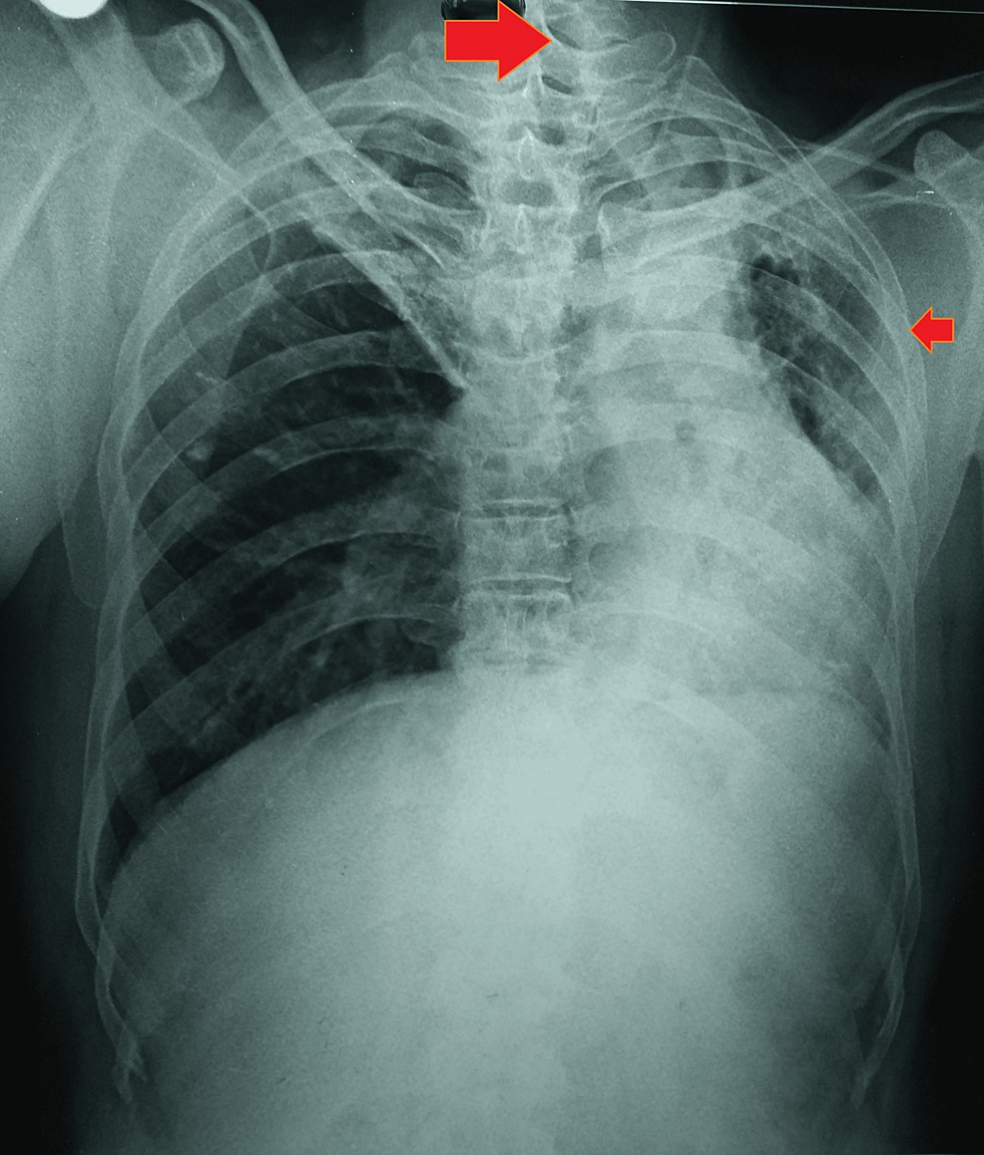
A plain chest radiograph suggestive of a destroyed left lung with tracheal deviation
